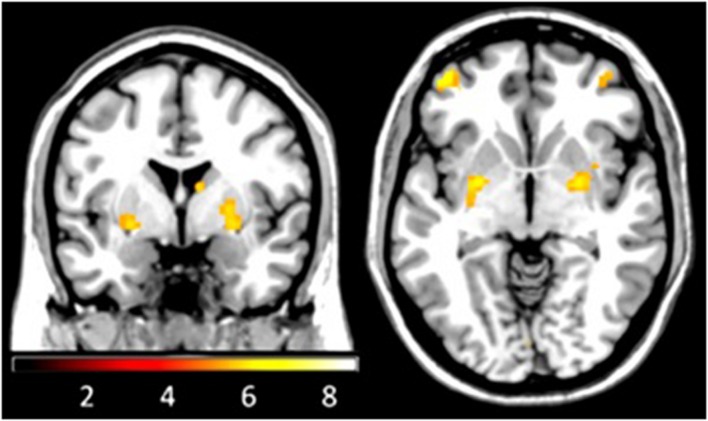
Functional magnetic resonance imaging during reversal learning. BOLD signal covaried with prediction errors in a network including the PFC, the parietal cortex, the striatum (putamen and caudate/thalamus-cluster) and the cerebellum (FWE-corrected on the whole brain level, Z-values > 4.8, *p*-values < 0.05).
